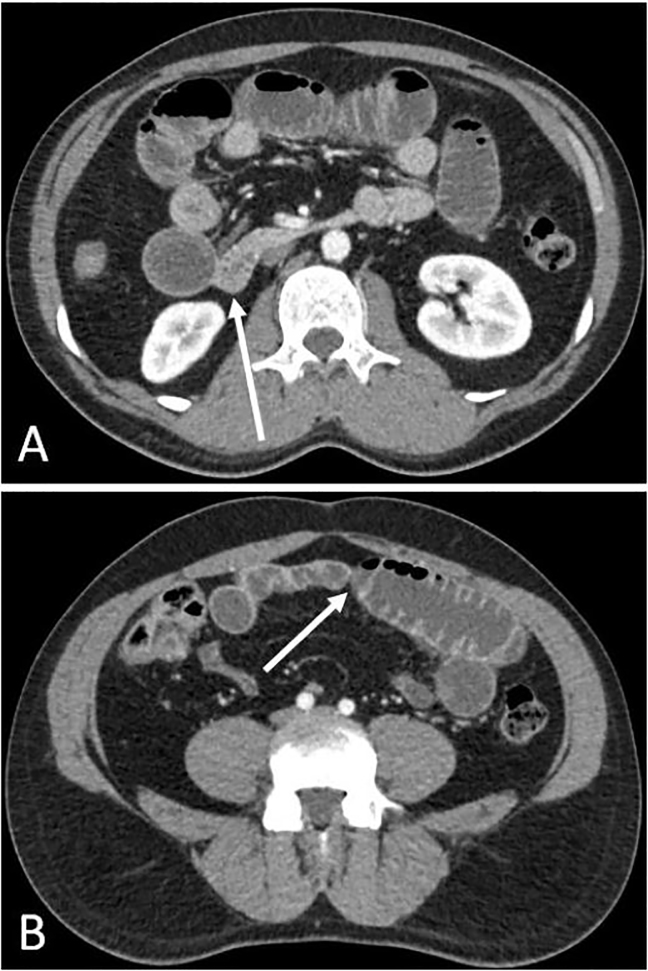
Axial view of abdominal computed tomography images showing the proximal (A) and distal (B) transition points (arrows) of a small bowel obstruction.
